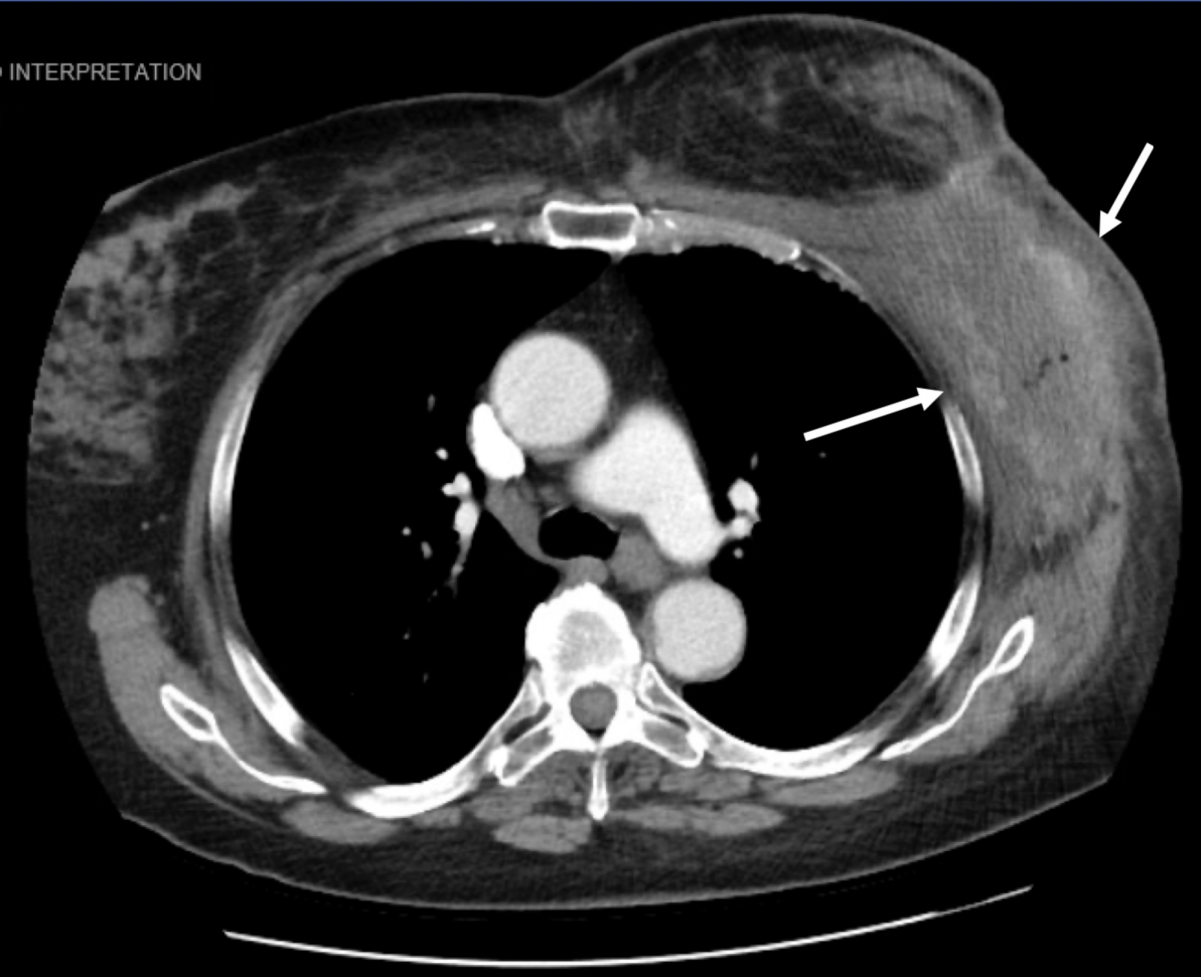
Axial CT scan showing a large, predominantly necrotic mass (white arrows) centered in the left lateral chest wall measuring approximately 9.8 x 5.6 cm CT: computed tomography
